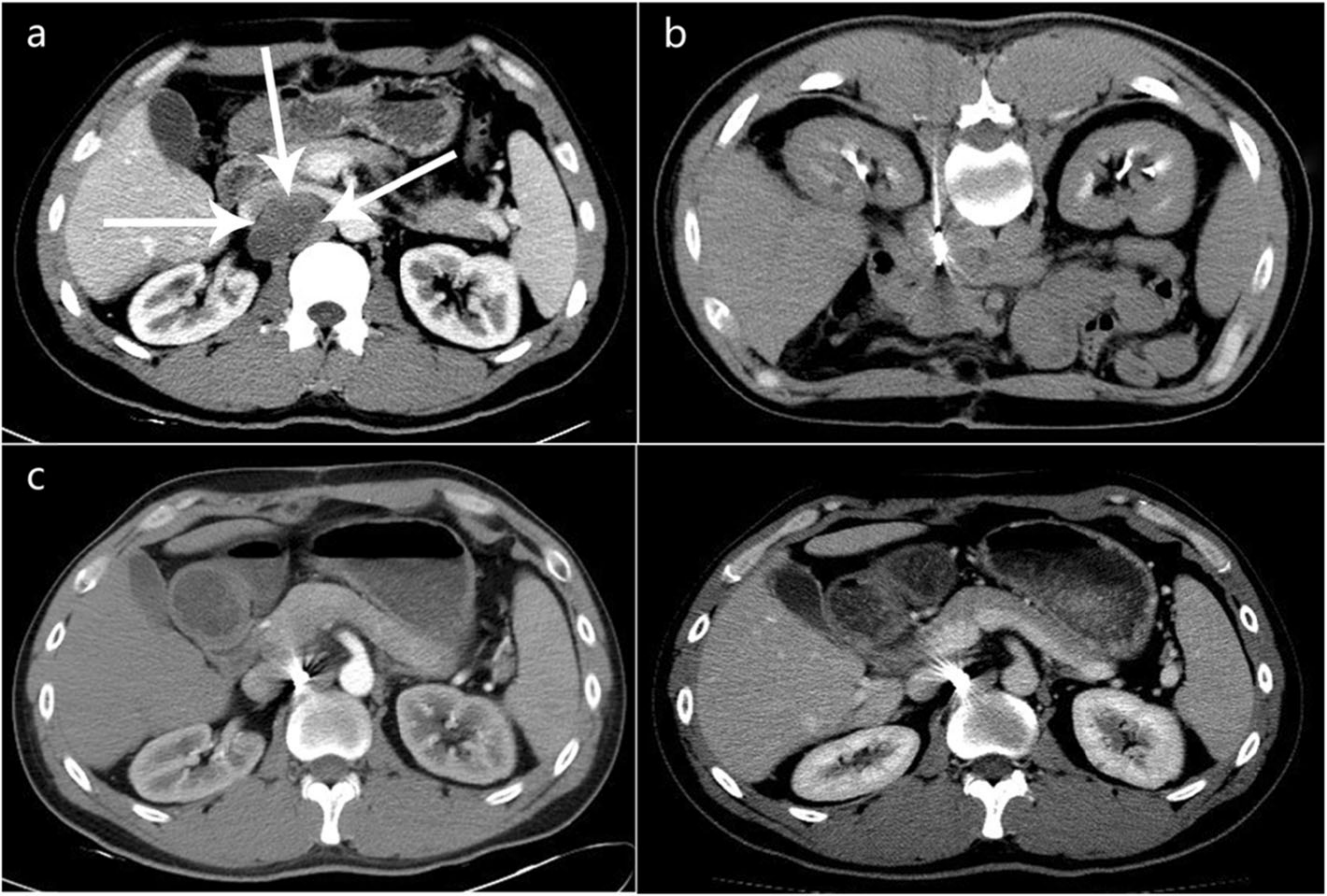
A 34-year-old male patient with retroperitoneal metastatic lymph nodes from primary hepato cellular carcinoma. a. Preoperative enhanced CT showed metastatic lymph nodes (arrow) with size of 42mm * 21mm, adjacent to the left renal vein, inferior vena cava, and abdominal aorta. b. Intraoperative CT scan. c, d. 4months after ^125^I brachytherapy, the lesion had disappeared.
